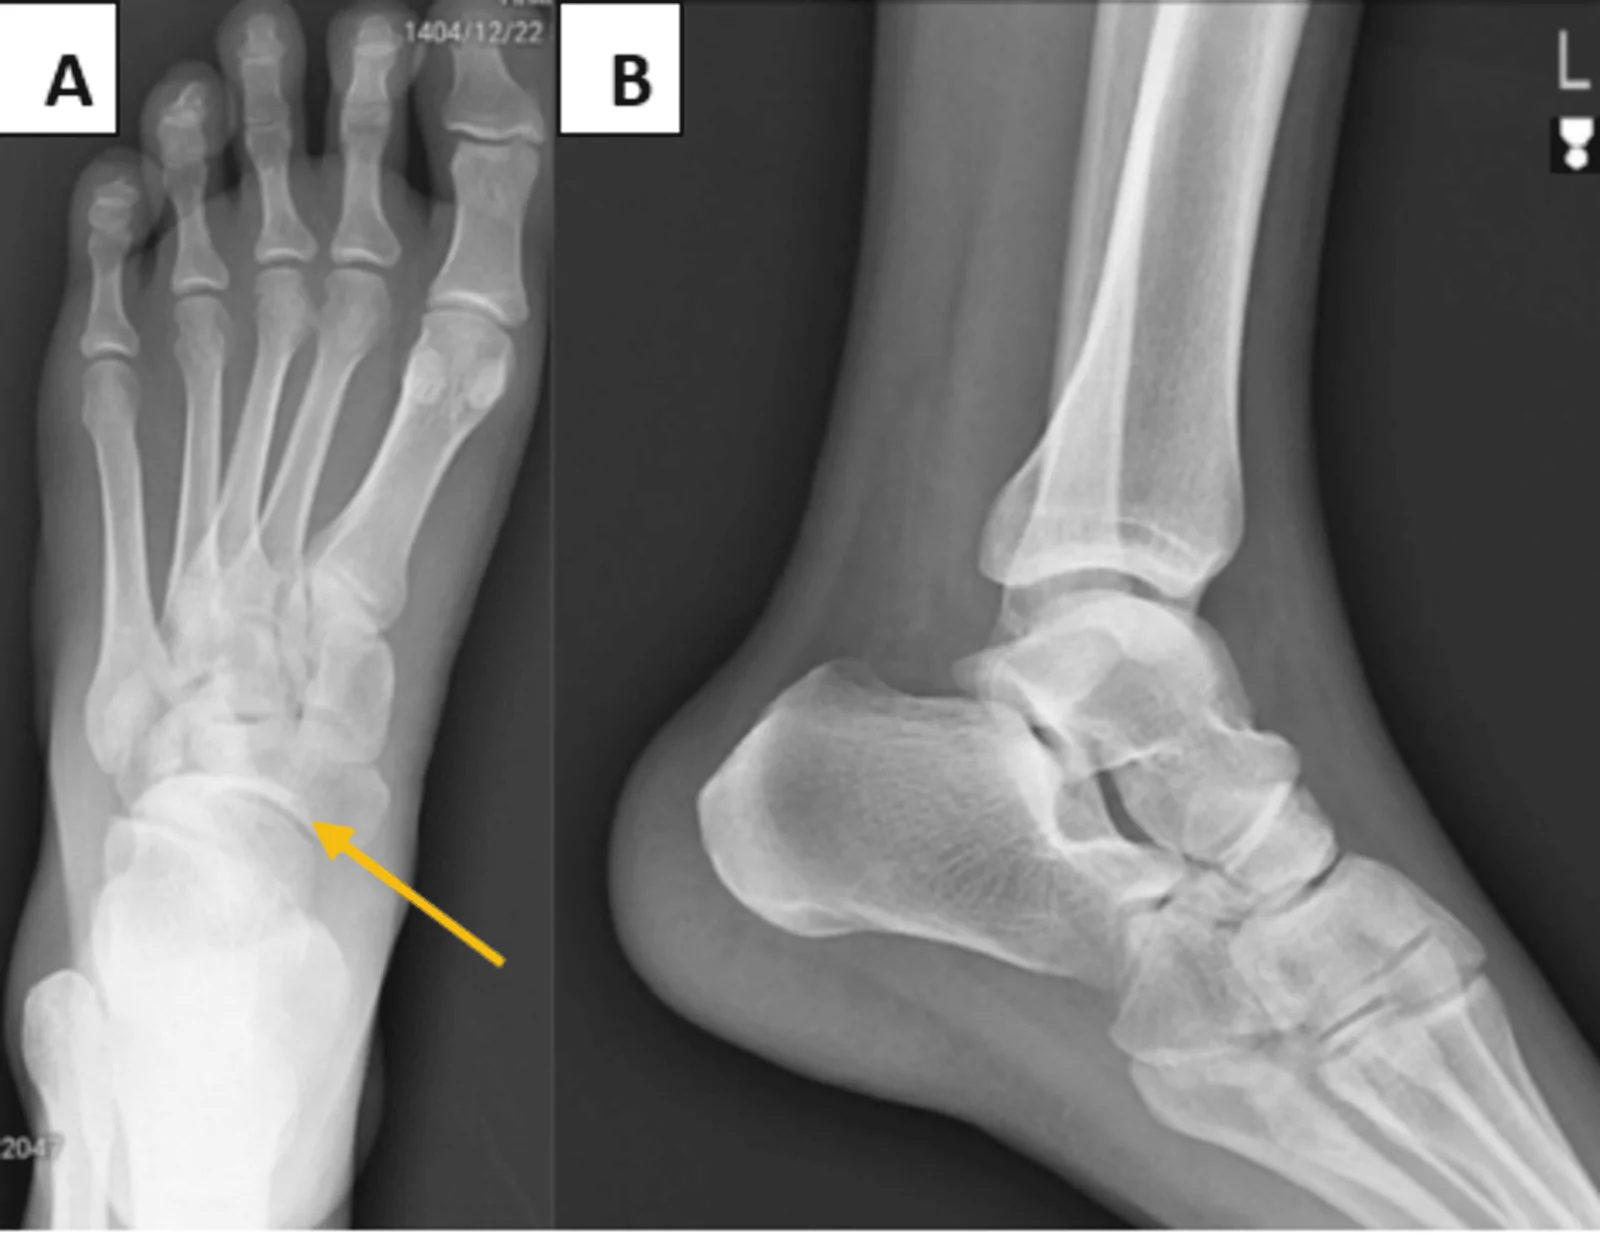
Preoperative radiographs taken 16 days after injury (A) Anteroposterior view and (B) lateral view (arrow showing left foot medial swivel injury)
